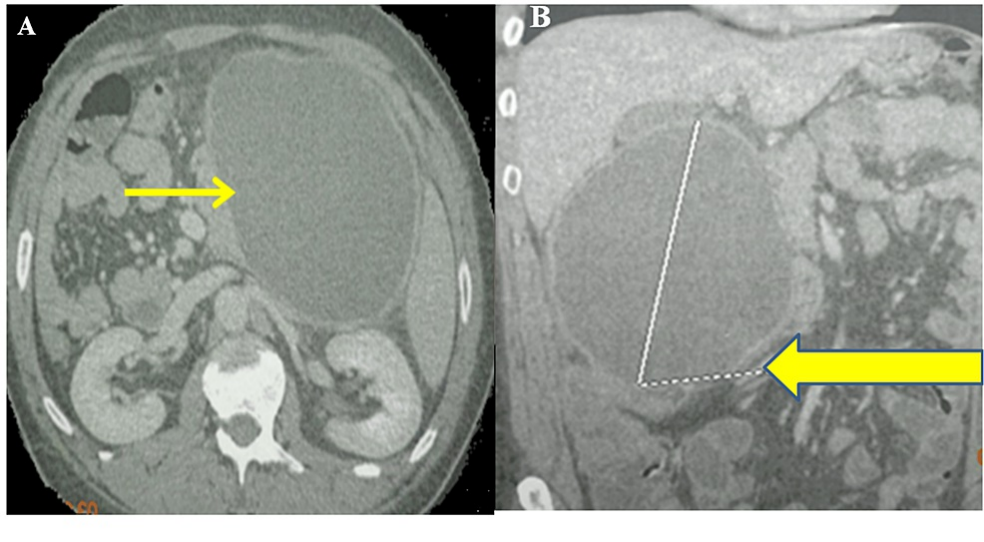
Contrast-enhanced computed tomography showing a round, well-circumscribed fluid collection of 17 x 10 cm (yellow arrow) on axial (A) and coronal sections (B).
